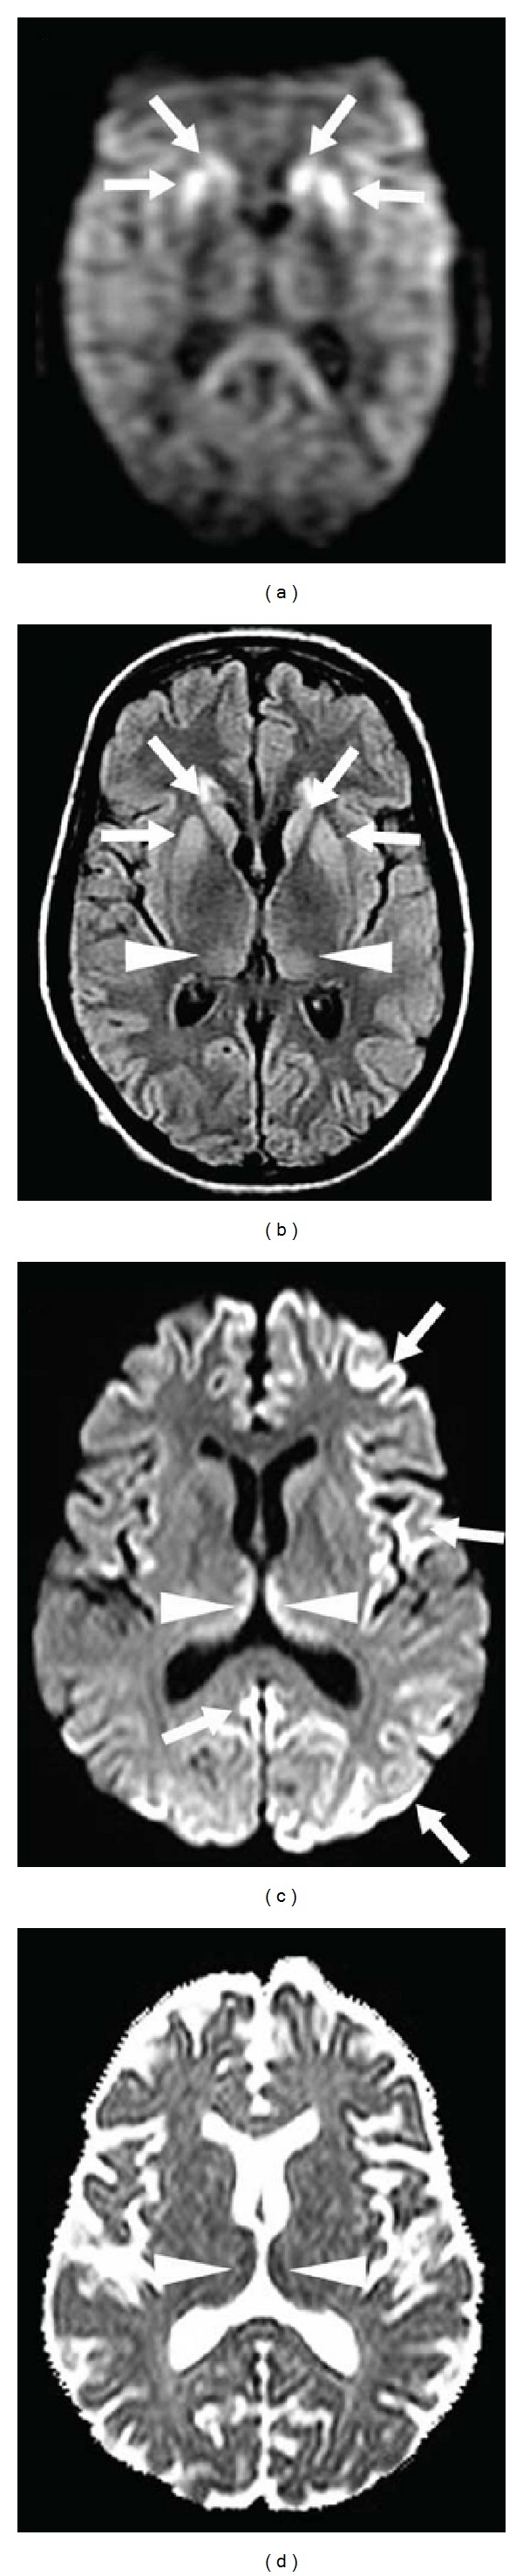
Two different sporadic CJD patients (patient 1, a and b; patient 2, c and d) showing in patient 1 bilateral caudate nucleus and putaminal (arrows) and to a lesser degree posteromesial and pulvinar thalamic (arrowheads) hyperintensities on DWI (a) and FLAIR imaging (b) and in patient 2 bilateral posteromesial thalamic (arrowheads) and multifocal cortical (arrows) hyperintensities on DWI (c), seen as hypointensity on ADC map (d). Anterior predominance of the thalamic involvement can be seen in patient 2.
